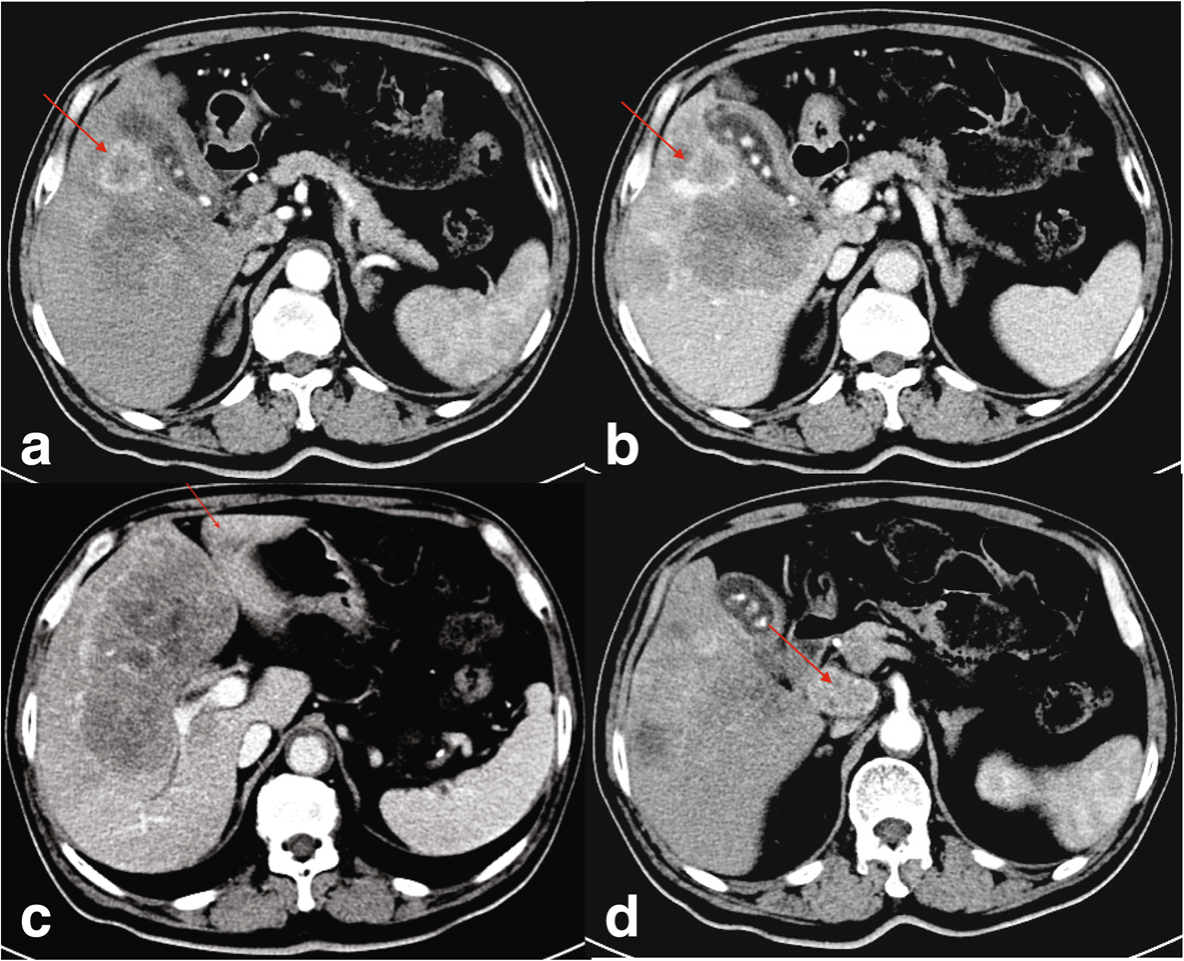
Enhanced-CT shows multiple lesions in the right and left hepatic lobes in different phases. The right hepatic lobe showed multiple patchy shadows (the largest 94 × 151 mm) and a circular enhanced lesion (arrow) in the (a) arterial and (b) venous phases. The left hepatic lobe showed a patchy shadow within the left lobe of liver (arrow) in (c) the venous phase. The portal triad showed an oval shadow (22 × 38 mm) with soft tissue density (arrow) in (d) the arterial phase
